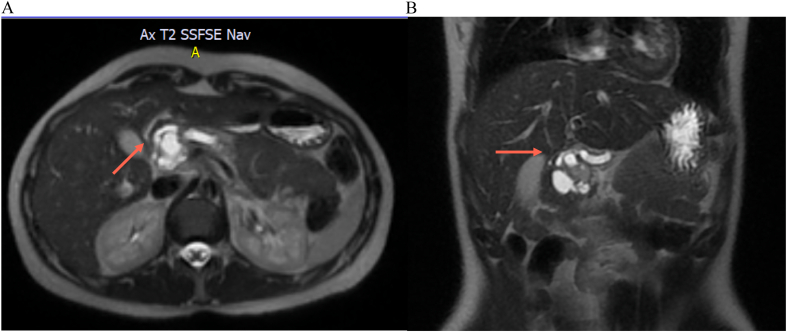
A: MRI in which we observe the Wirsung dilatation and the atrophy of the pancreatic body and tail. B: MRI in which we observe the Wirsung dilatation and the atrophy of the pancreatic body and tail.
